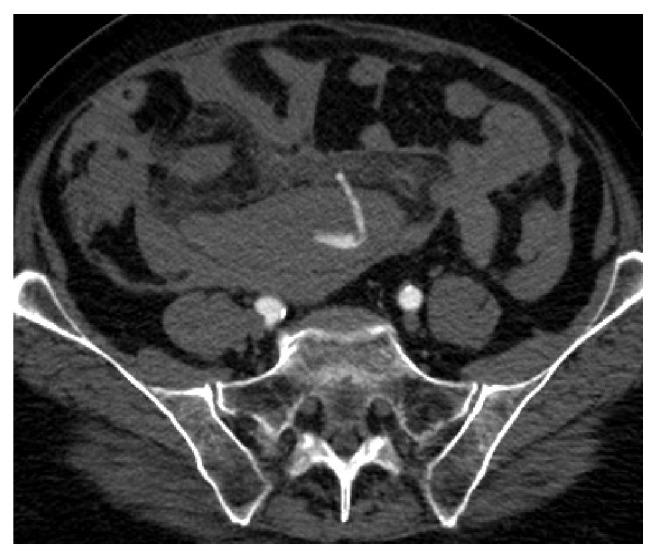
CTA abdomen hyperdense hemorrhaging vessel.
